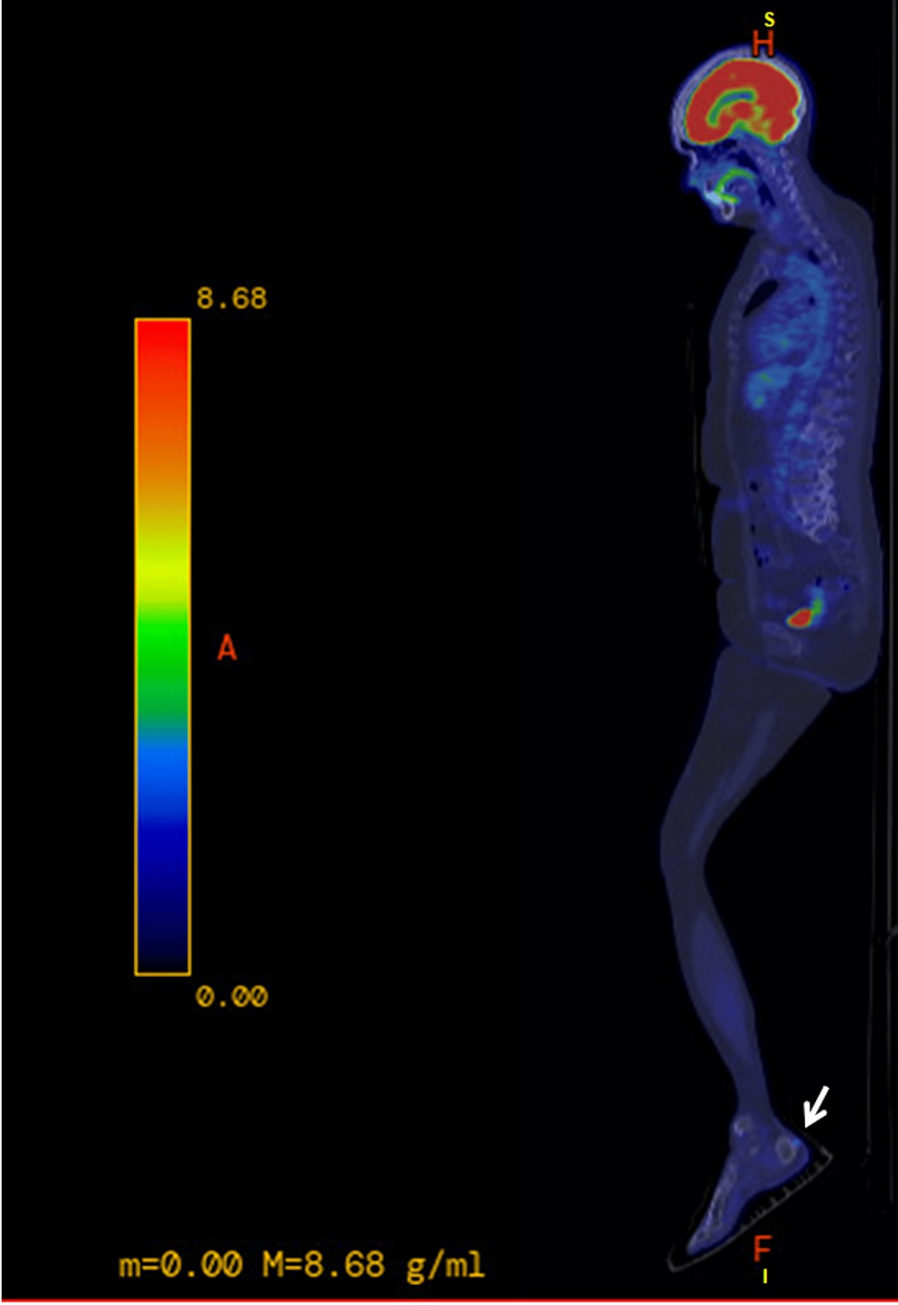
PET-CT scan results noting focal uptake of the left heel just medial to midline PET-CT: positron emission tomography-computed tomography
